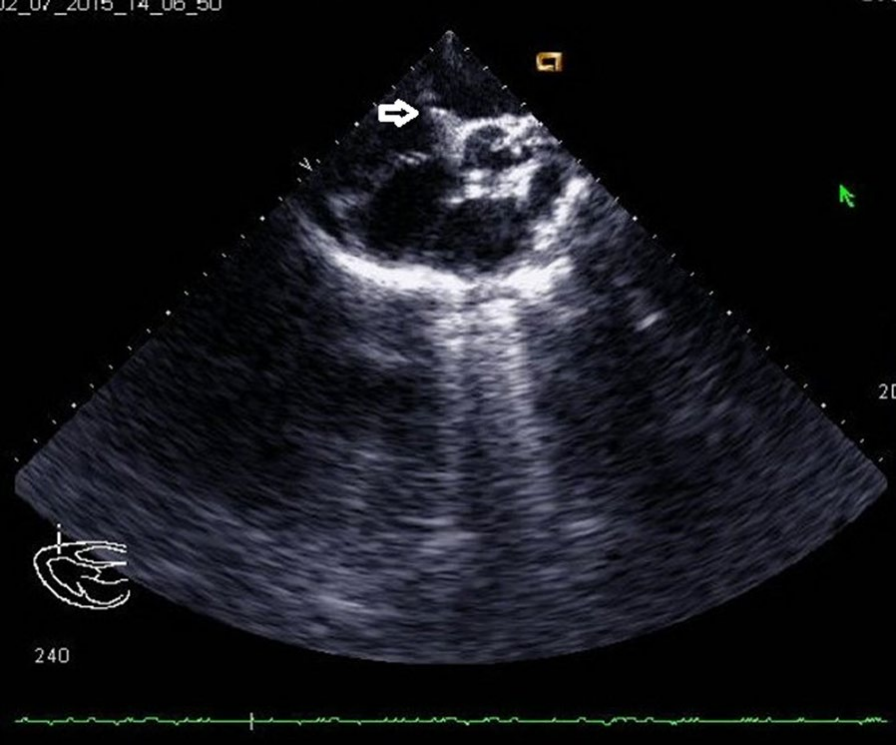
2Dechocardiography parasternal short axis view showing double chambered right ventricle (DCRV), *white arrowhead* showing the band dividing the ventricular cavity
